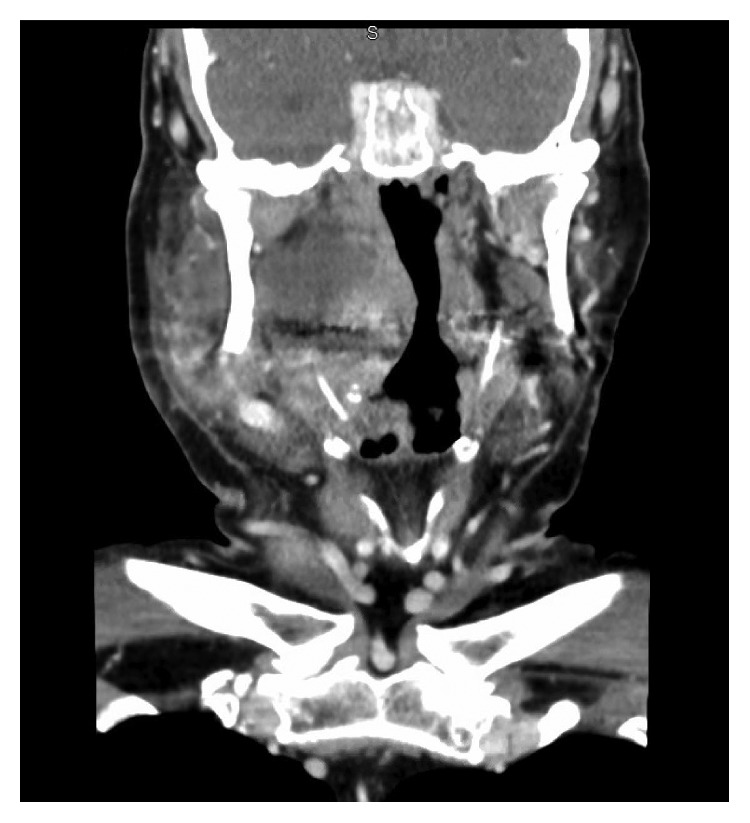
Coronal CT image depicting deep lobe parotid fluid collection extending to the prestyloid parapharyngeal space.
